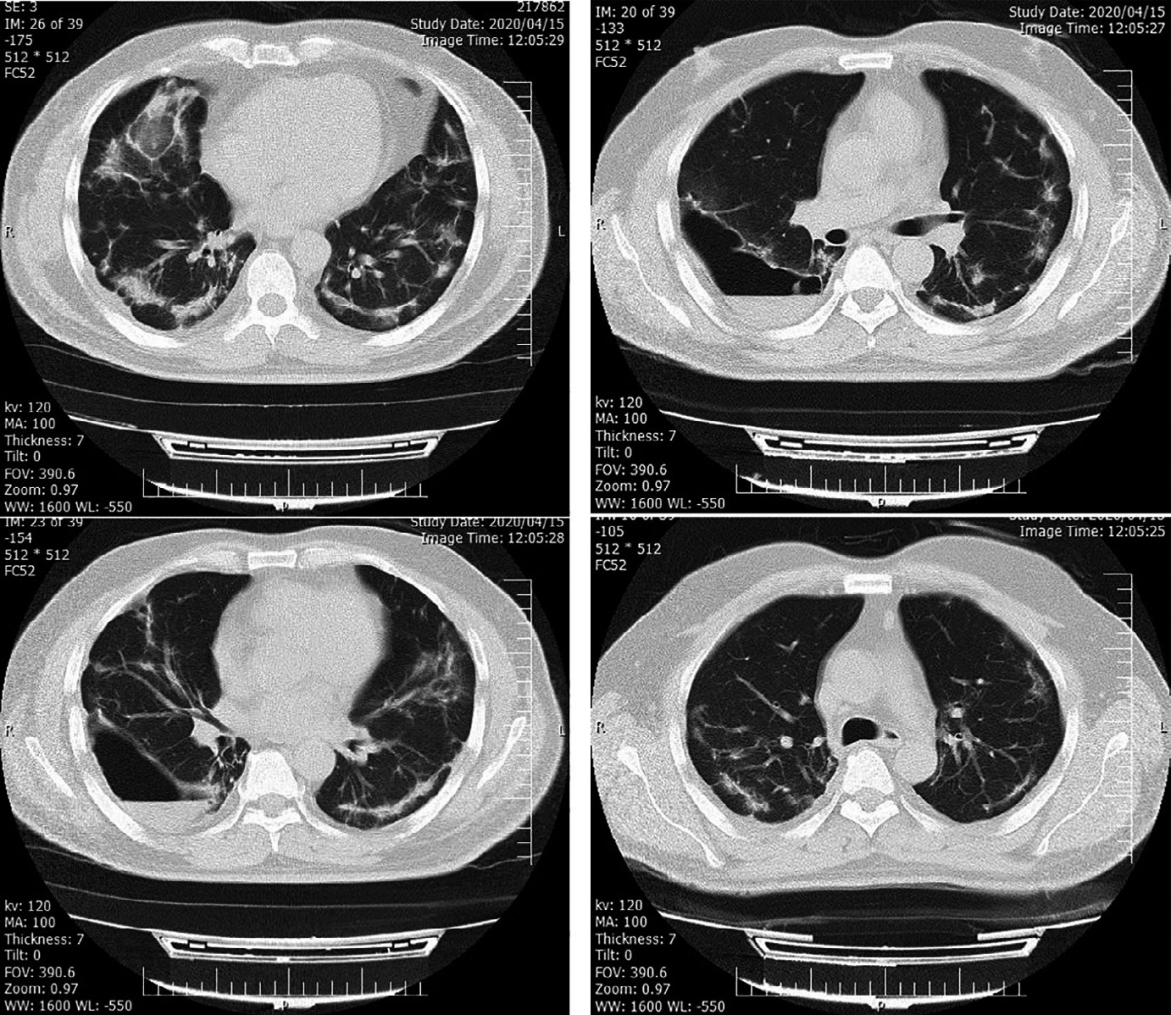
Chest CT scan with lung abscess
